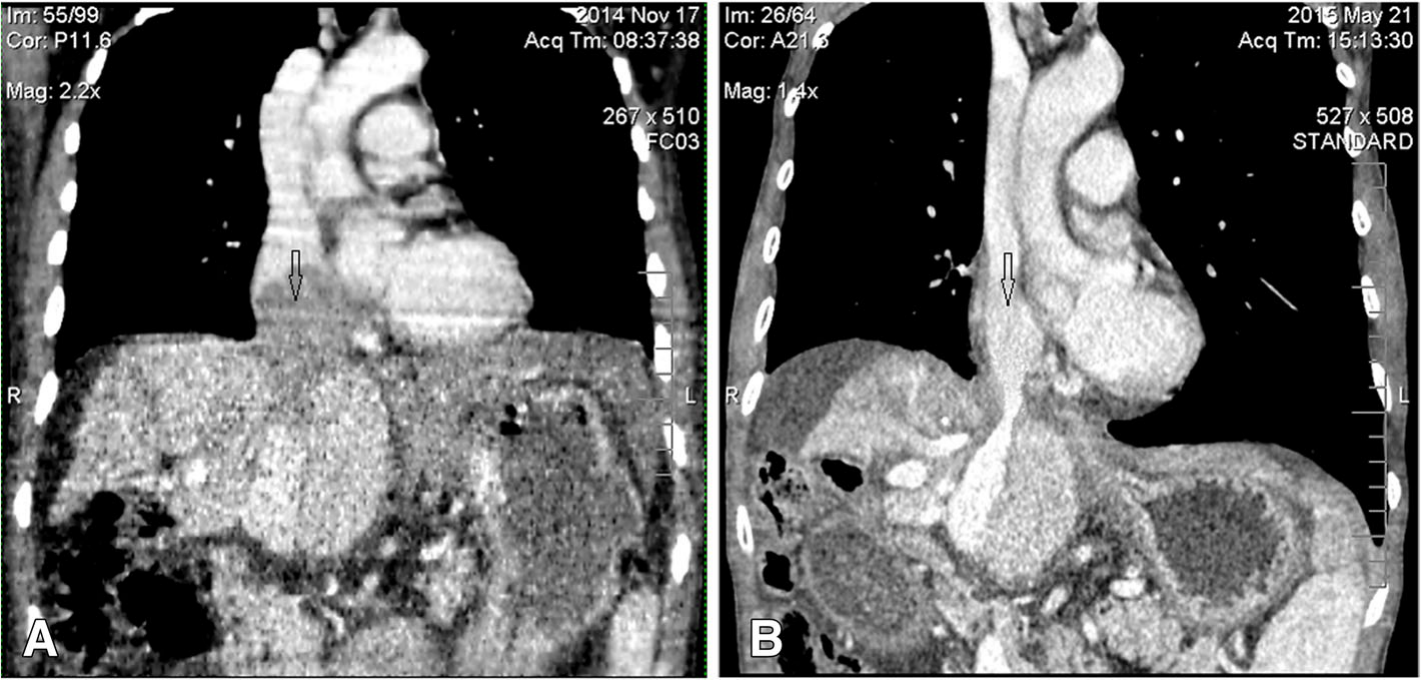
Computed tomography (CT) follow-up images of one RA TT case. a Before HFRT, the TT (7.5*6.5 cm) filled the right atrium, resulting in circulatory disorders and cardiac enlargement. b 6 months after completion of RT, the TT disappeared and circulation returned to normal
